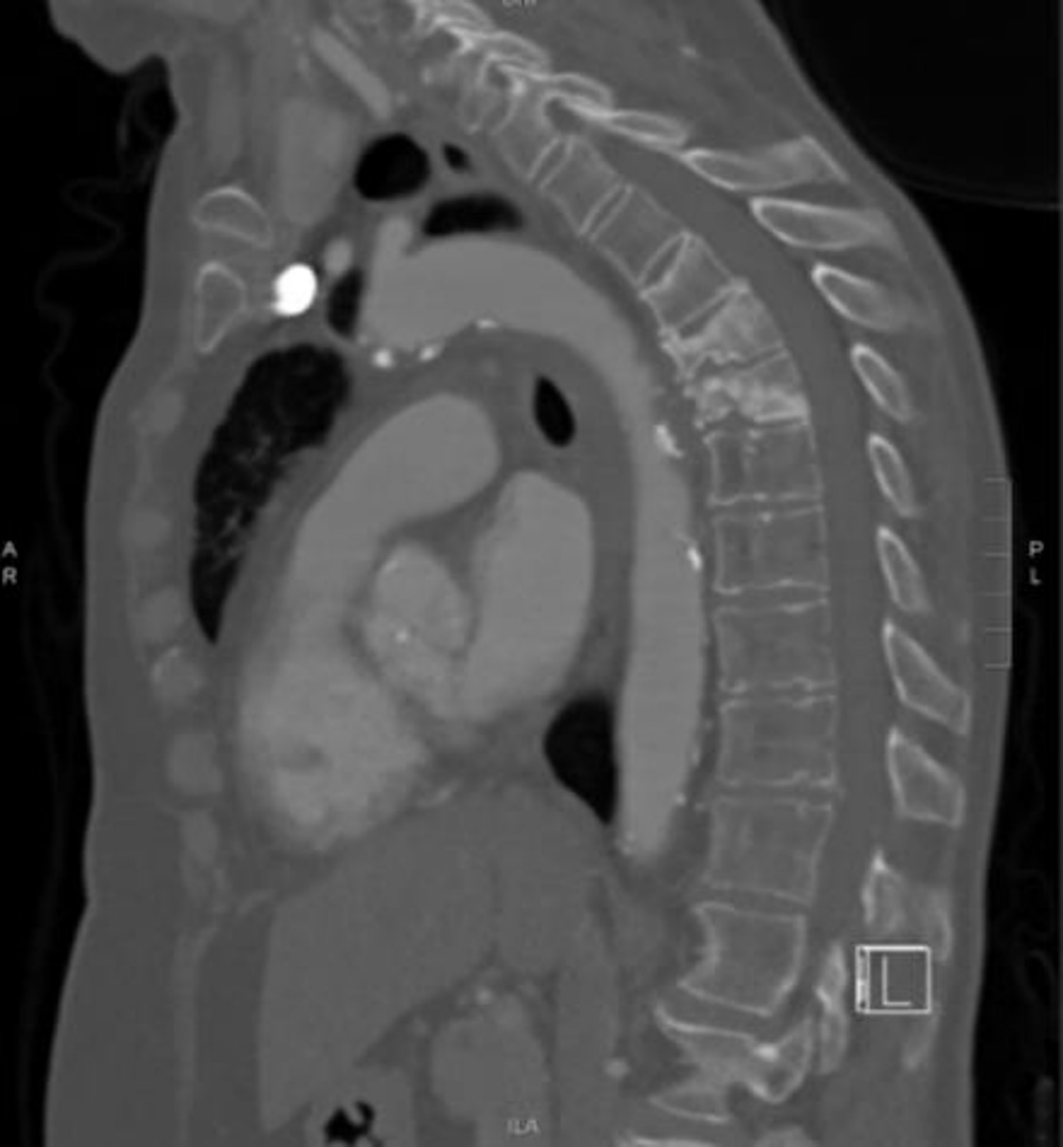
Sclerotic pathological wedge compression fractures of T6 and T7 secondary to metastatic disease on sagittal reconstruction of staging CT thorax in patient with primary non-small cell lung cancer correlating with uptake in bone scan in Figure 5.
